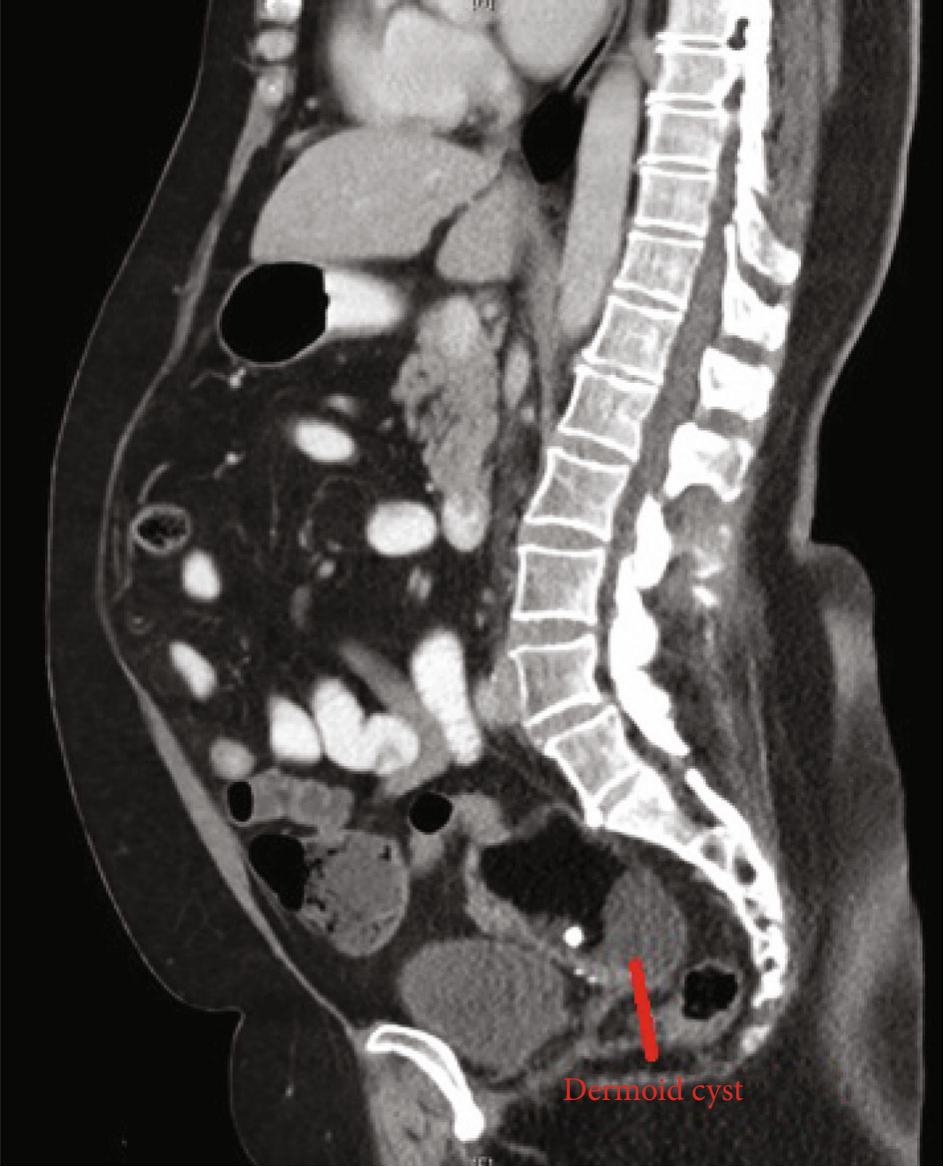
Sagittal view of CT abdo-pelvis showing a likely dermoid cyst in the right adnexa.
